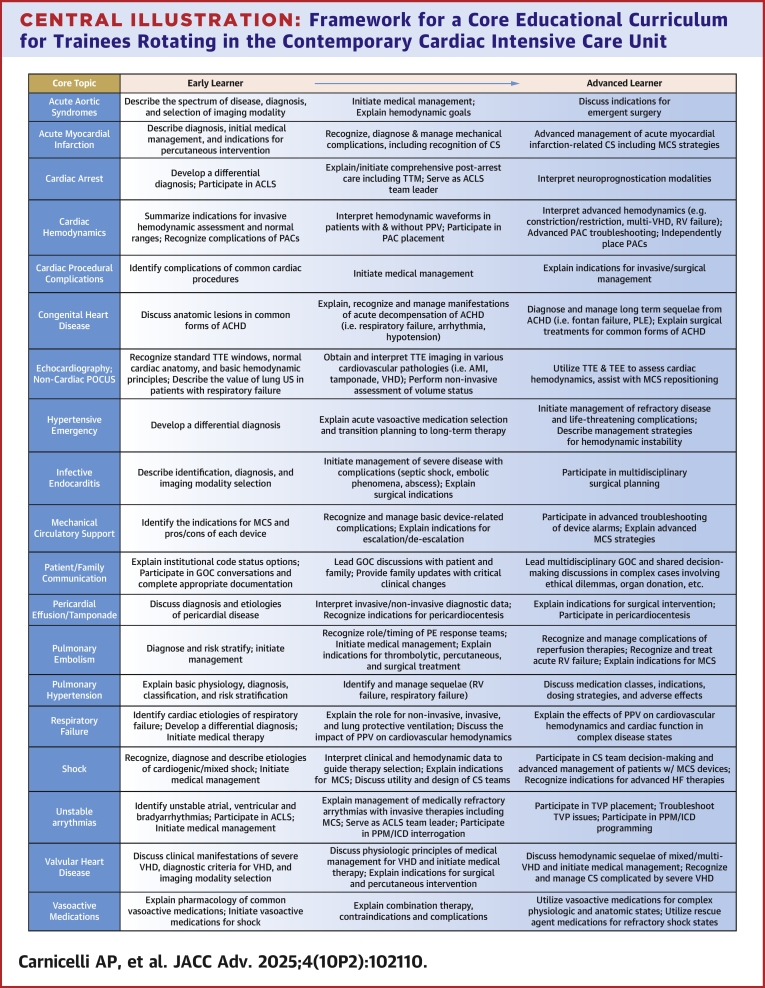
Framework for a Core Educational Curriculum for Trainees Rotating in the Contemporary Cardiac Intensive Care Unit ACHD = adult congenital heart disease; ACLS = advanced cardiovascular life support; AMI = acute myocardial infarction; CS = cardiogenic shock; GOC = goals of care; ICD = implantable cardioverter defibrillator; MCS = mechanical circulatory support; PAC = pulmonary artery catheter; PE = pulmonary embolism; PLE = protein-losing enteropathy; POCUS = point of care ultrasound; PPM = permanent pacemaker; PPV = positive pressure ventilation; RV = right ventricle; TEE = transesophageal echocardiography; TTE = transthoracic echocardiography; TTM = targeted temperature management; TVP = transvenous pacemaker; US = ultrasound; VHD = valvular heart disease
